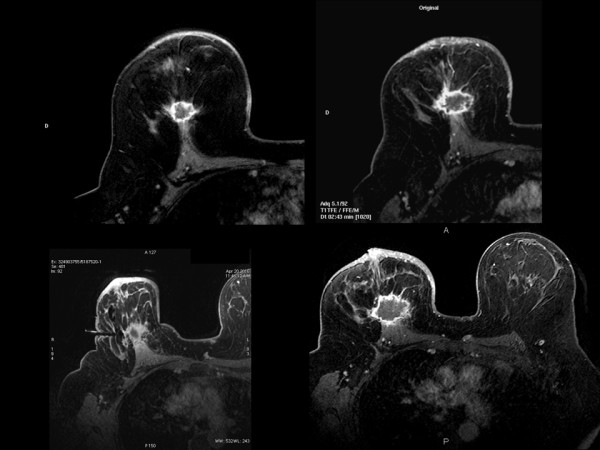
Series of contrast-enhanced MRI shows a ring enhancement of the mass. The feature is significantly associated with triple-negative breast cancer. In the lower right corner, MRI-guided vacuum-assisted biopsy that failed to obtain malignant tissue.
